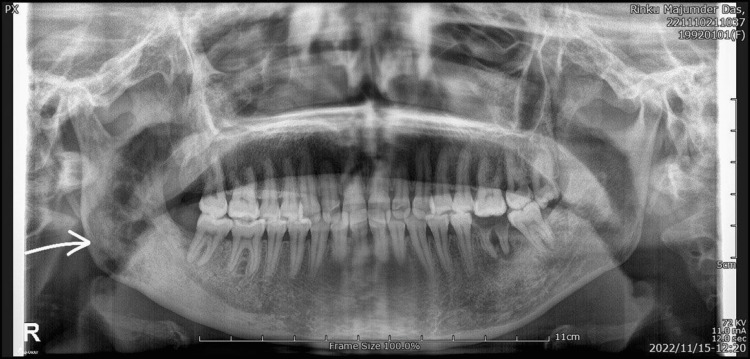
Pre-operative orthopantomogram showing bony changes in the ramus on the right side of the mandible
